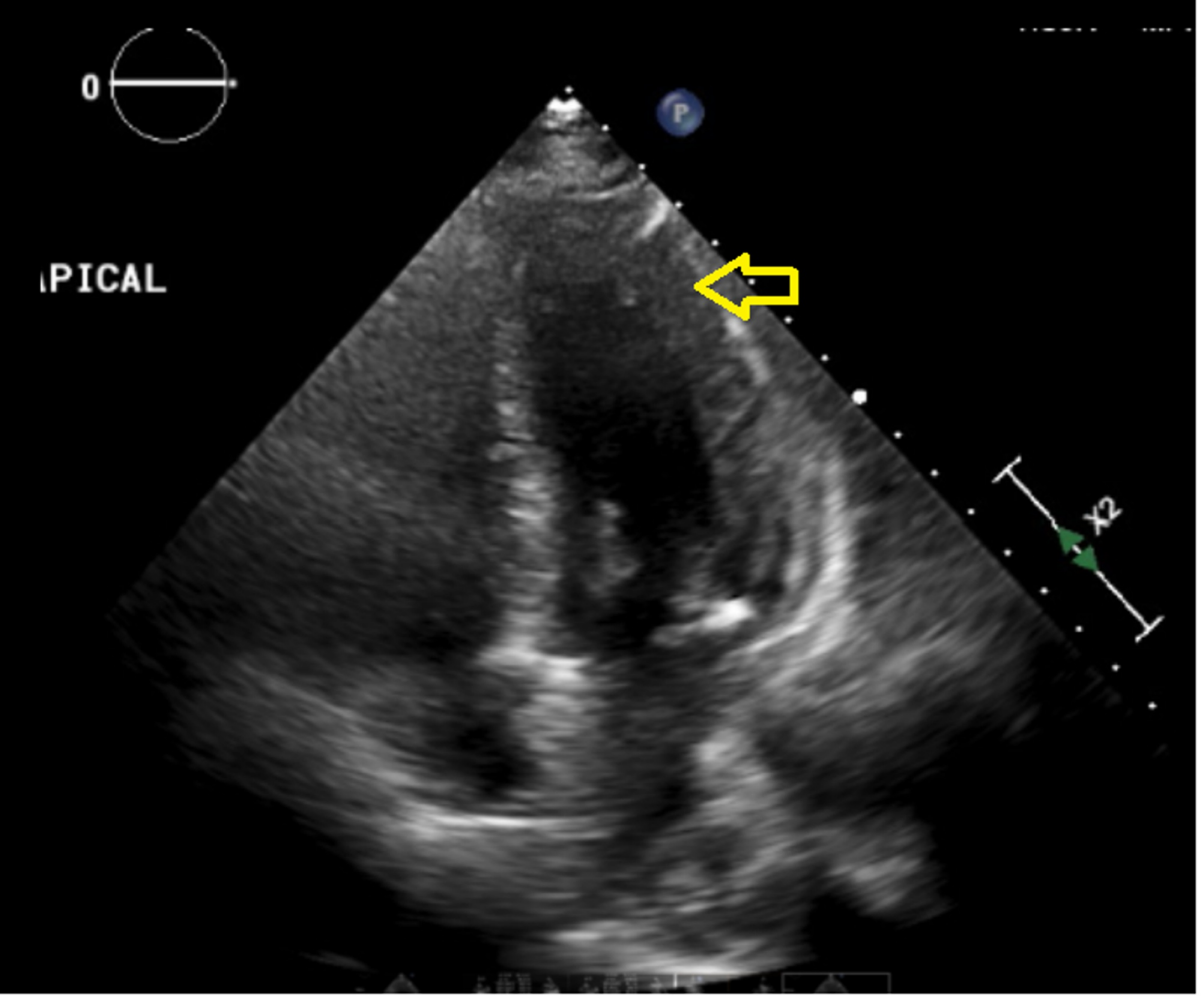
Cardiac echocardiogram Apical view: moderate concentric left ventricular hypertrophy (yellow arrow).
